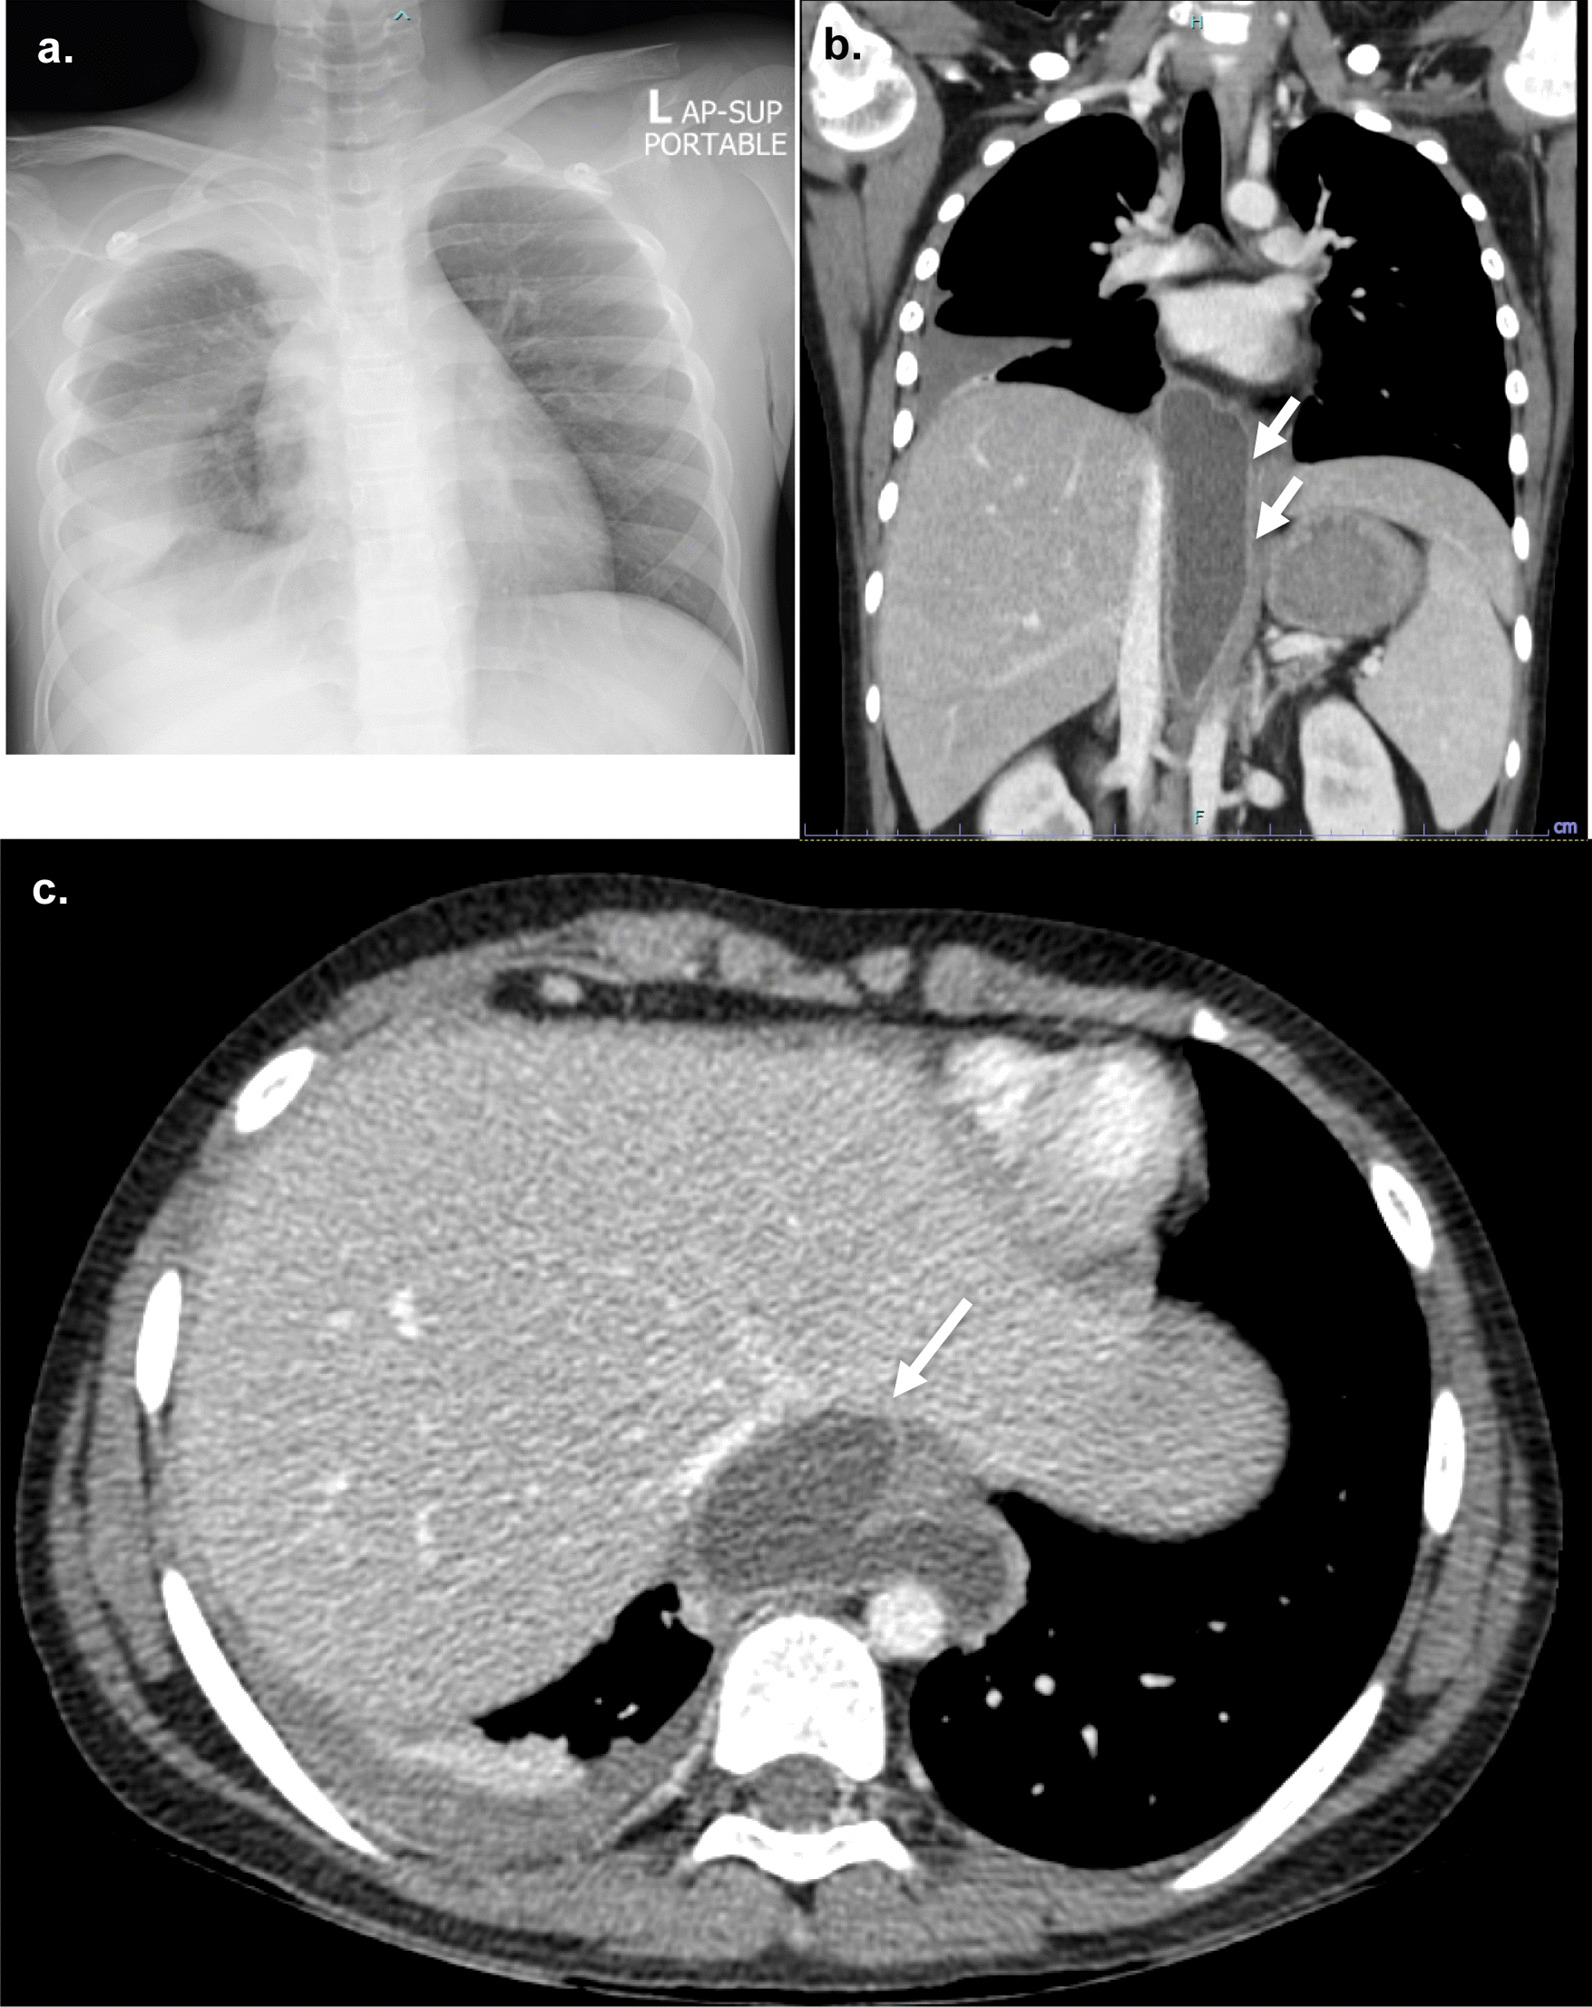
Chest radiography and CT scan from the second admission a massive pleural effusion, b transverse and c coronal plane of CT abdomen demonstrate a mediatinal pseudocyst and PPF arise from proximal pancreatic duct (arrow) reaching up to right pleural cavity
